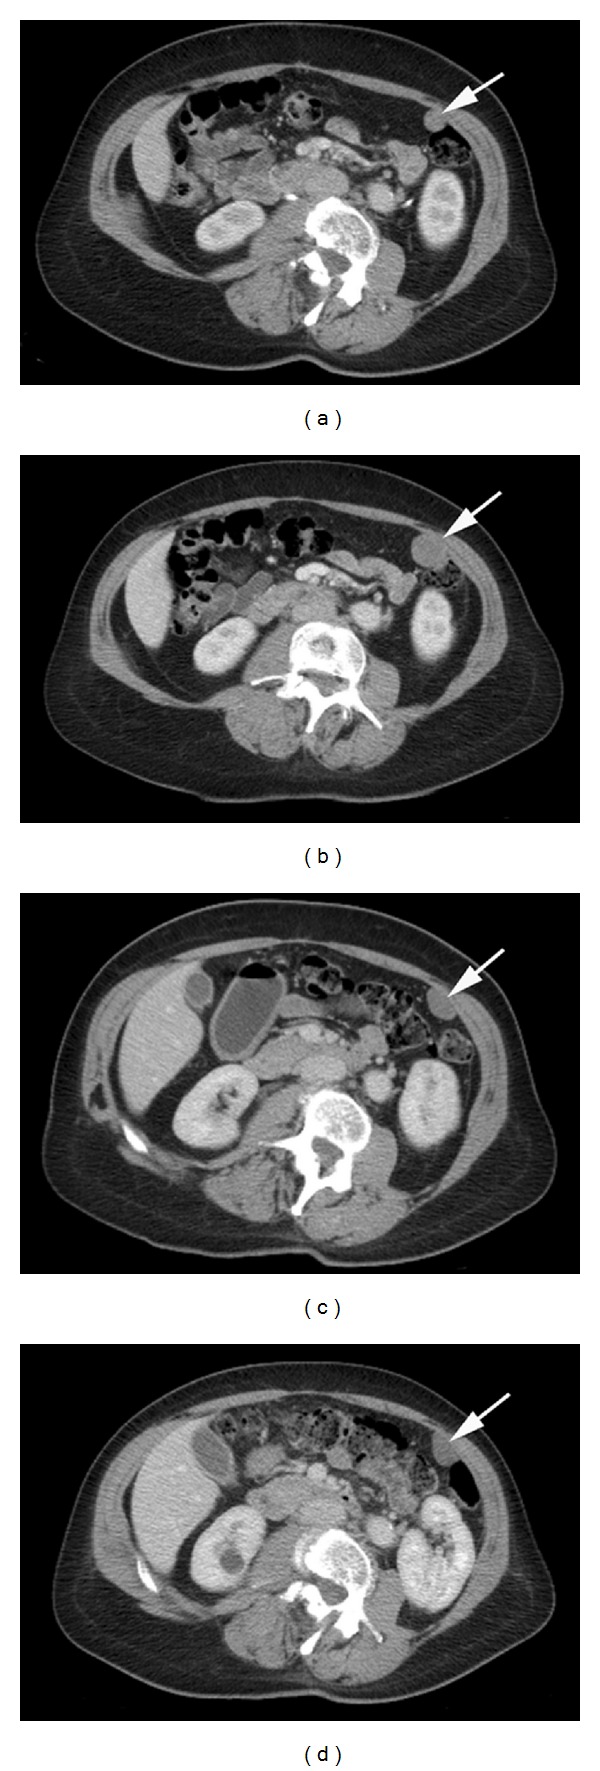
Axial contrast enhanced CT images show a peritoneal deposit within the left side of the abdomen (arrows). Prior to commencing sirolimus, the deposit progressed by RECIST 1.1 over a period of 6 months ((a) and (b)). CT staging at 4 months (c) and 13 months (d) on treatment with sirolimus showed that the deposit had reduced in size but was within the limits of stable disease by RECIST v1.1. A further pelvic deposit (not shown) also reduced in size but overall disease remained stable by RECIST v1.1. However, assessment by Choi criteria which incorporates attenuation changes classified disease status as partial response at 4 months and further partial response at 13 months.
